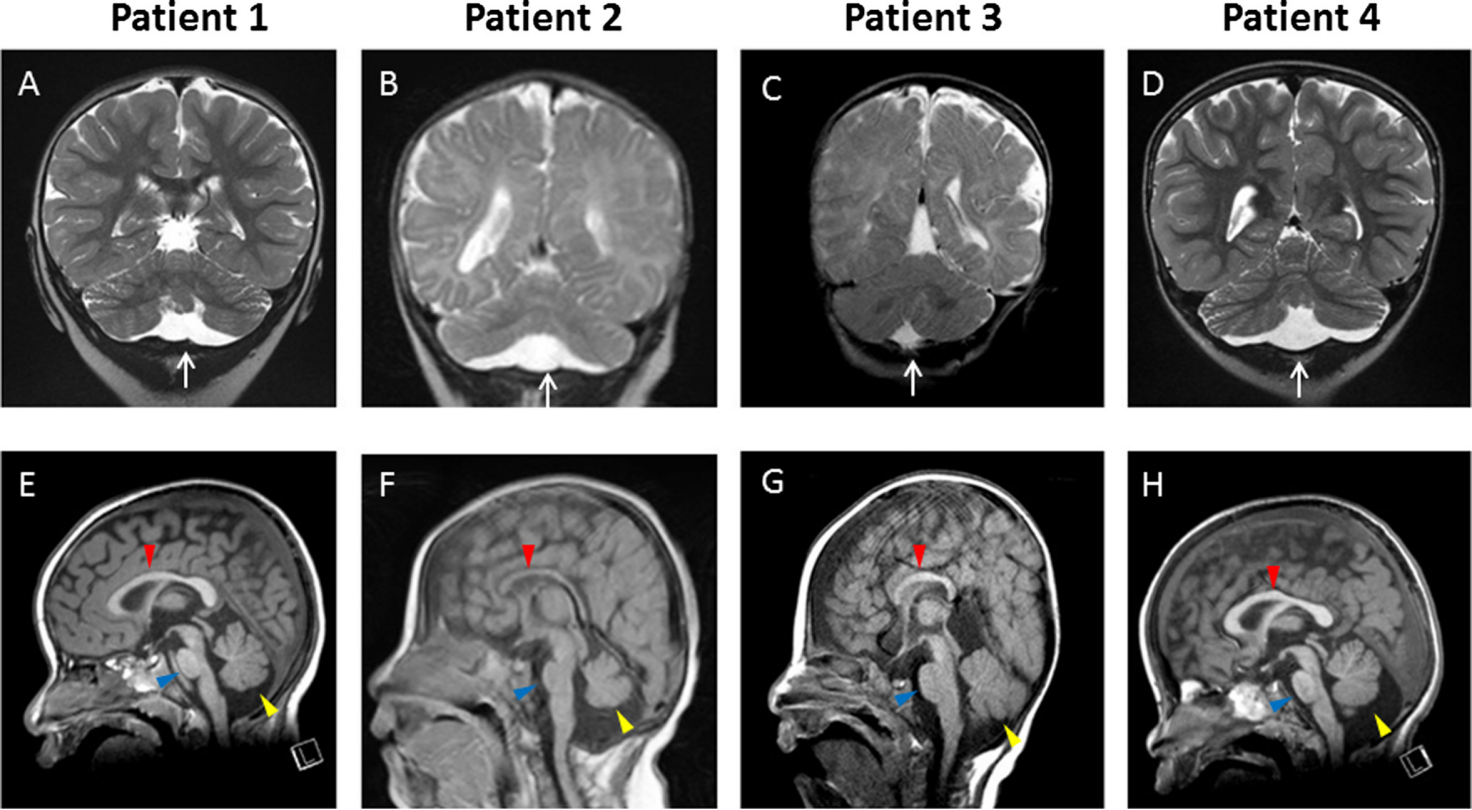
Selected coronal and sagittal MR images in four patients. (A and E) Patient 1: A 3‐year‐old female with 2p15p16.1 deletion (3.24 Mb). The cranial MRI was performed at 2 years of age. (A) T2‐weighted coronal and (E) T1‐weighted sagittal MR images show (A) mild hypoplastic, flattened cerebellar hemispheres with proportionally reduced size of the vermis and dilated subarachnoid space beneath the cerebellum (white arrow), and (E) mild hypoplasia of the pons (blue arrowhead), a slightly small cerebellar vermis (yellow arrowhead), and intact corpus callosum (red arrowhead). (B and F) Patient 2: A 22‐month‐old female with 2p15p16.1 deletion (5.04 Mb). The cranial MRI was performed at 9 months of age. (B) T2‐weighted coronal and (F) T1‐weighted sagittal MR images show (B) hypoplastic, flattened cerebellar hemispheres with proportionally reduced size of the vermis with dilated subarachnoid space beneath the cerebellum (white arrow), and (F) prominent hypoplasia of the pons (blue arrowhead), very small cerebellar vermis (yellow arrowhead), and thin corpus callosum (red arrowhead). (C and G) Patient 3: A 4 years 2 months old male with 2p14p16.1 deletion (5.12 Mb). The cranial MRI was performed at 5 months of age. (C) T2‐weighted coronal and (G) T1‐weighted sagittal MR images show (G) normal size and shape of the cerebellar hemispheres, cerebellar vermis (yellow arrowhead), pons (blue arrowhead), and small corpus callosum (red arrowhead), and (C) without dilated subarachnoid space beneath the cerebellum (white arrow). (D and H) Patient 4: A 5‐year‐old male with 2p16.1 deletion (1.12 Mb). The cranial MRI was performed at 2 years of age. (D) T2‐weighted coronal and (H) T1‐weighted sagittal MR images show (D) mild hypoplastic, flattened cerebellar hemispheres with proportionally reduced size of the vermis with dilated subarachnoid space beneath the cerebellum (white arrow), and (H) mild hypoplasia of the pons (blue arrowhead), small cerebellar vermis (yellow arrowhead), and intact corpus callosum (red arrowhead).
